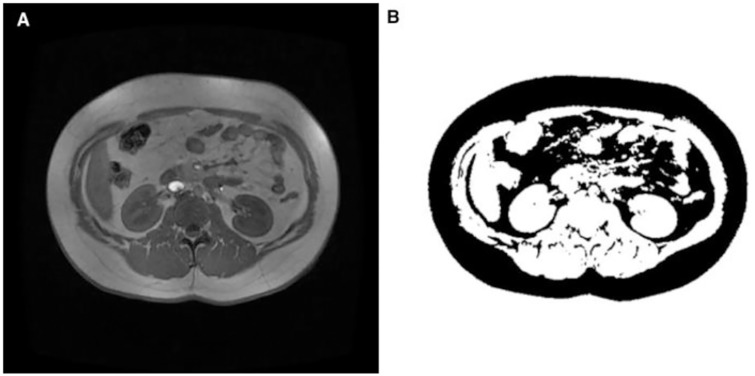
Magnetic resonance image of obese male (BMI 32.59). A) .jpg MRI image from L3-L4. B) Binary ImageJ image for measurement of fat: fat appears in black. Image shows intra-abdominal (visceral) and subcutaneous fat.
